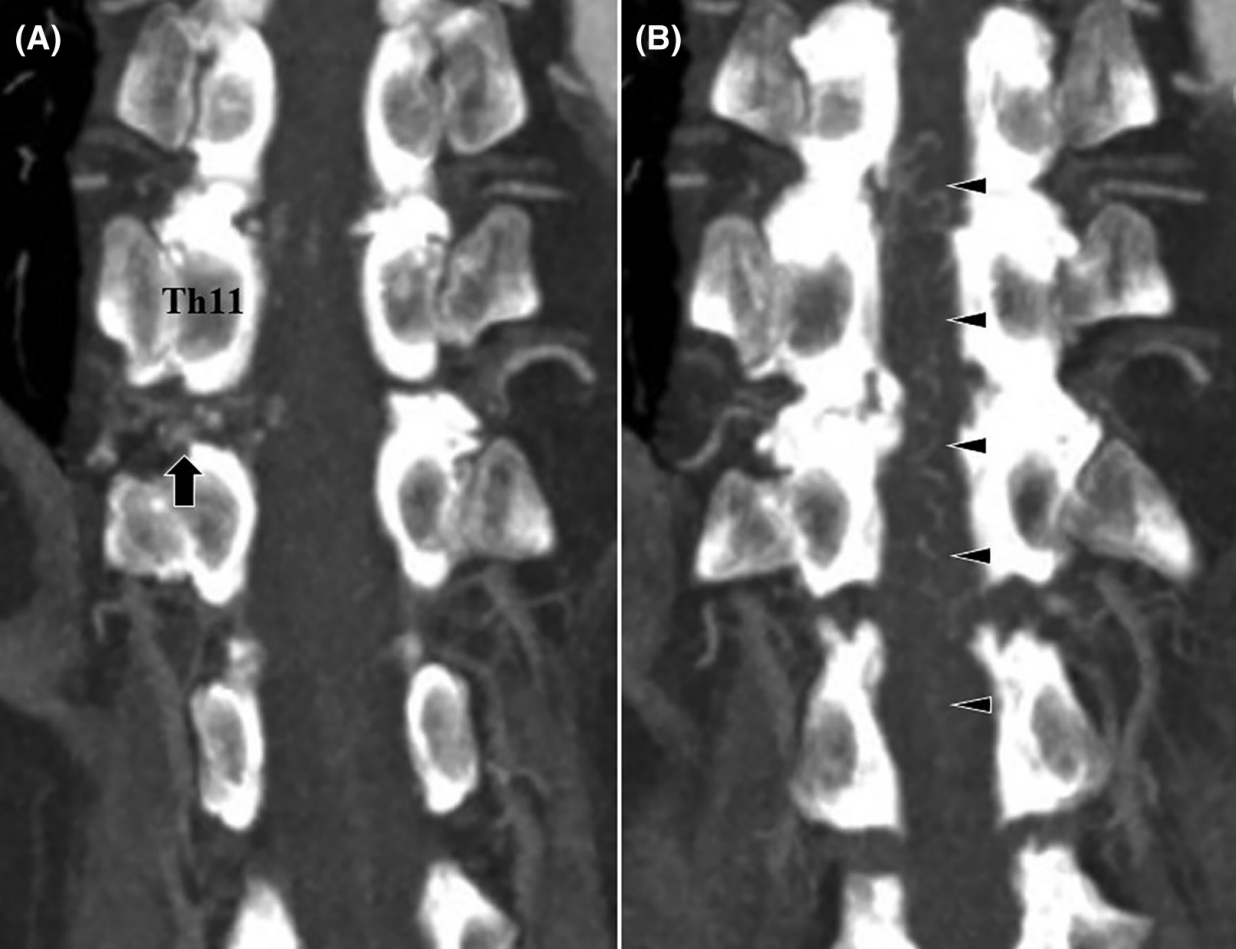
Preoperative computed tomography (CT) scan. (A, B) Coronal CT showing a premedullary vessel at the level of Th11/12 and a dilated premedullary drainage vein.
